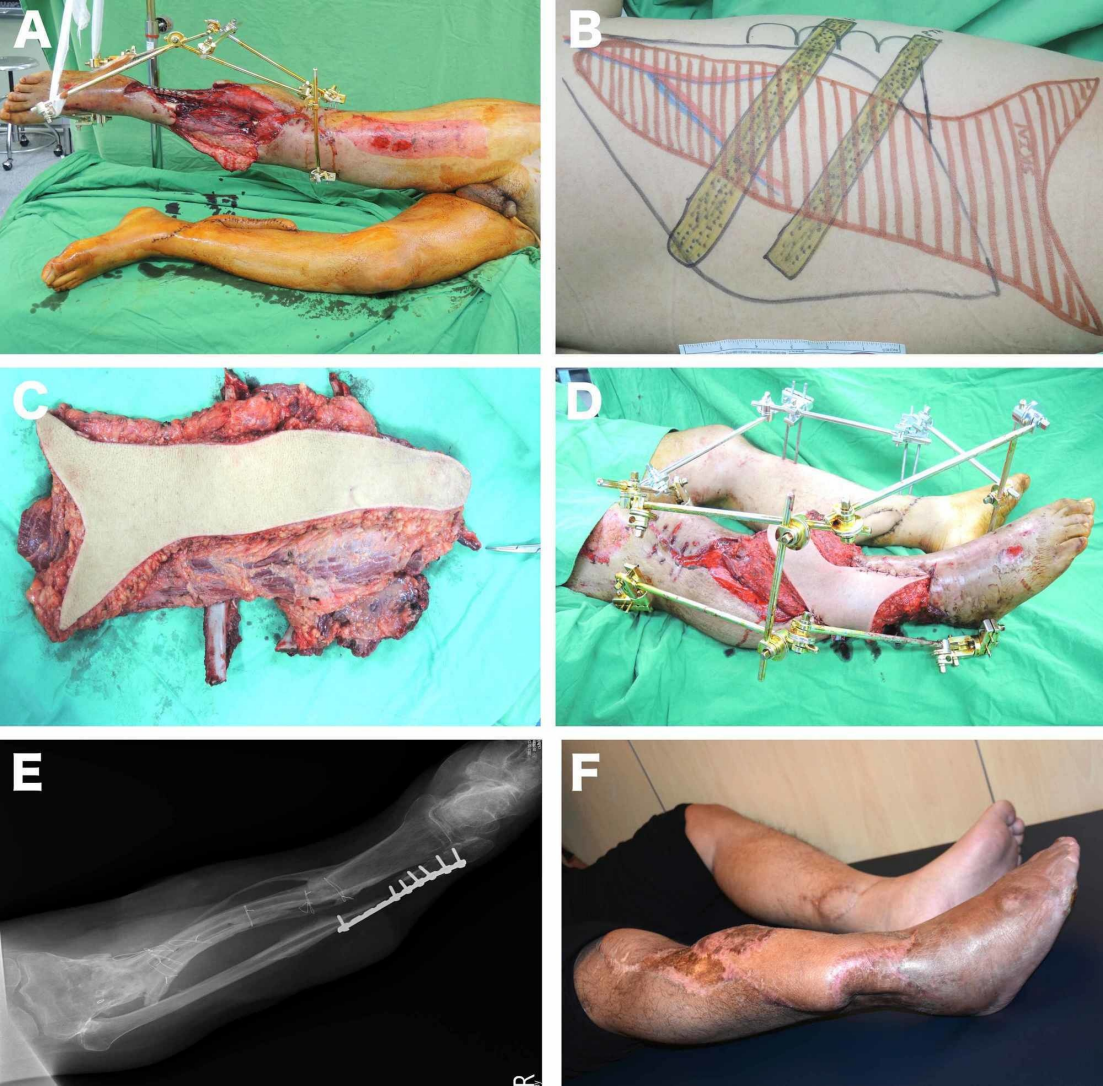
Lower extremity reconstruction with free extended fleur-de-lis LD osteomyocutaneous flap (A) A 26 x 17 cm tissue defect on the right leg due to injury sustained in a motor vehicle accident; (B) The design of extended fleur-de-lis LD osteomyocutaneous flap involving 7th and 9th ribs (the vertical dimension of the flap is 35 cm and the horizontal dimension of the extension is 15 cm); (C) Harvested flap; (D) Flap inset; (E) X-ray, at one-year postoperative, showing the bony integration; (F) The appearance of the leg at the three-year postoperative evaluation.
